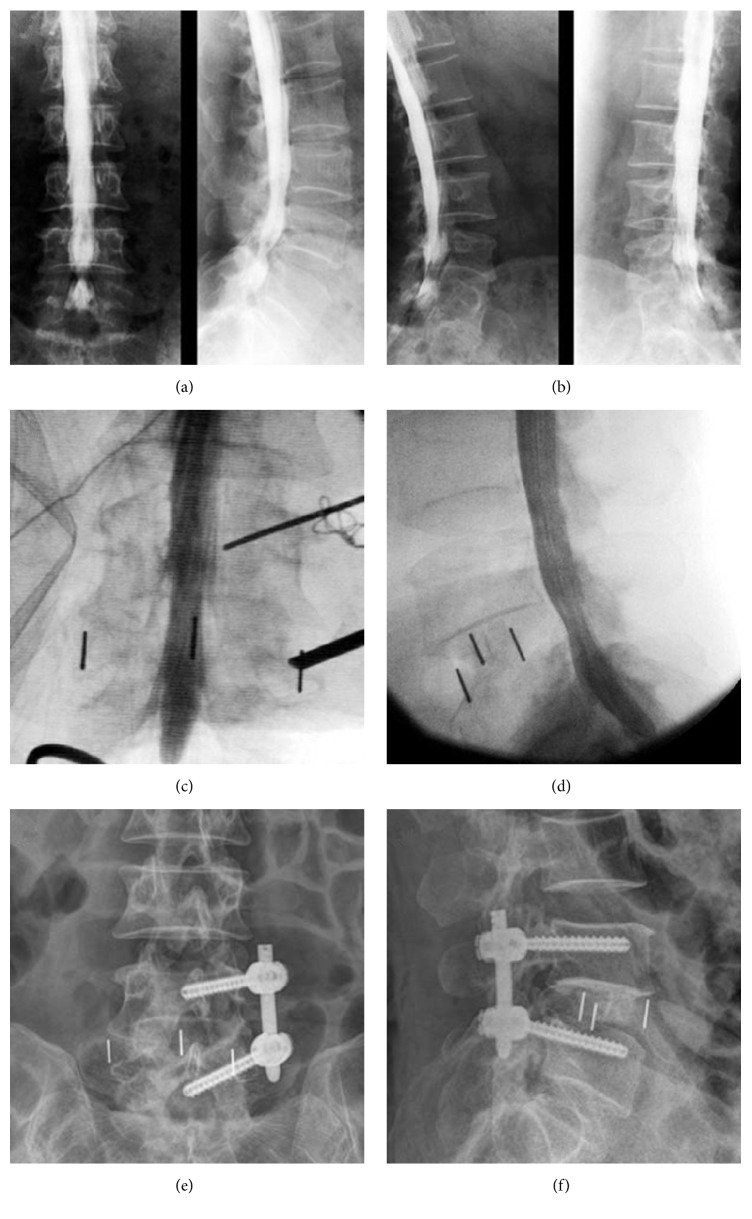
Radiographs of Case (B)*: preoperative myelography indicated canal stenosis of L4-5 level ((a) and (b)). Intraoperative myelography during LLIF demonstrated resolution of filling defect ((c) and (d)). Postoperative lumbar plane images showed disc height increase at L4-5 level ((e) and (f)).
